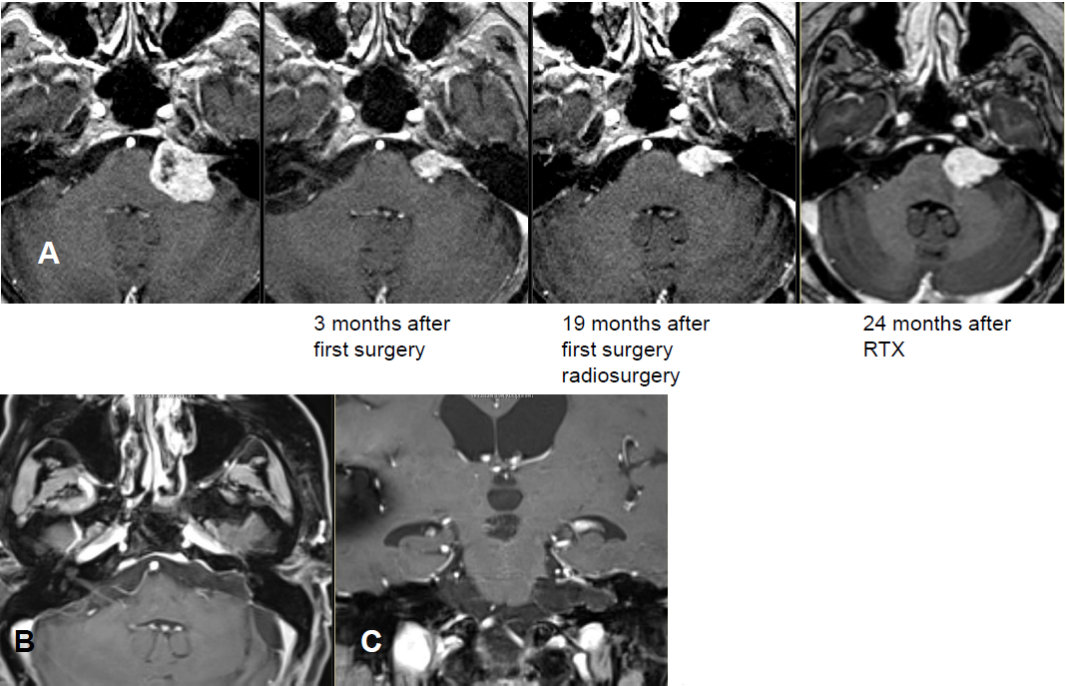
Upper row: preoperative MRI (T1-w + CM; A transversal) and follow-up MRI. 3 months after the 1^st^ surgery, there is still a larger residual tumor in situ. After 19 months is has slightly increased, also 2 years after irradiation, the tumor is progressive, the brainstem is compressed. Lower row: MRI 6 months after the 2^nd^ resection. T1-w + contrast agent application; B transversal; C coronal.
